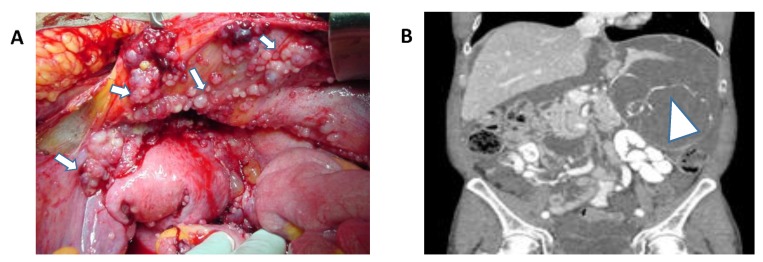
(A) Abdominal peritoneal metastasis from ovarian cancer. White arrows demonstrate peritoneal disease (Permission granted by Scott D Richard). (B) Coronal CT-scan of the abdomen and pelvis depicting extensive peritoneal metastasis and tumor burden from appendiceal cancer. White arrow demonstrates extensive intraperitoneal disease.
